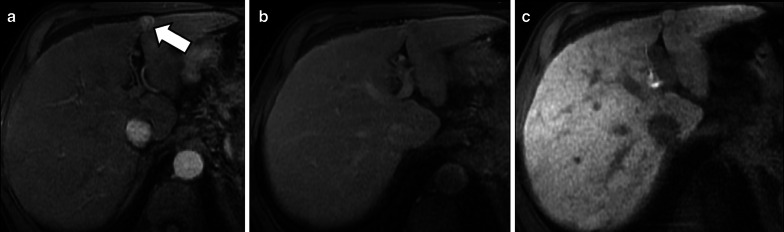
A 65-year-old patient with HCV-related cirrhosis and hepatocellular carcinoma. Gadobenate dimeglumine-enhanced MRI shows an HCC with (a) arterial phase hyperenhancement (arrow) in the arterial phase, (b) non-peripheral washout in the portal venous phase and (c) iso-hyperintensity in the hepatobiliary phase surrounded by a non-enhancing capsule
